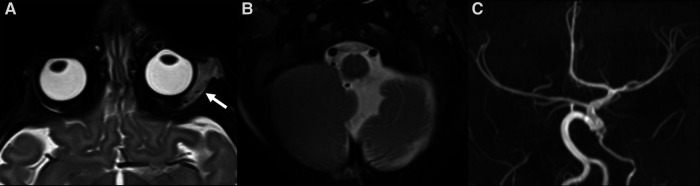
MRI of 4-month-old girl with a red mass of the left orbit. Axial T2 weighted images (A,B) show a left orbital hyperintense mass (big white arrow in A) with tiny flow-voids involving the extra and intraconal compartments, consistent with infantile hemangioma (IH); on the same sequence the cerebellar atrophy on the ipsilateral side of the IH is also noted (B). Additionally, MR TOF-3D disclosed abnormalities of the intracranial circulation (C). The diagnosis of PHACES was finally made.
